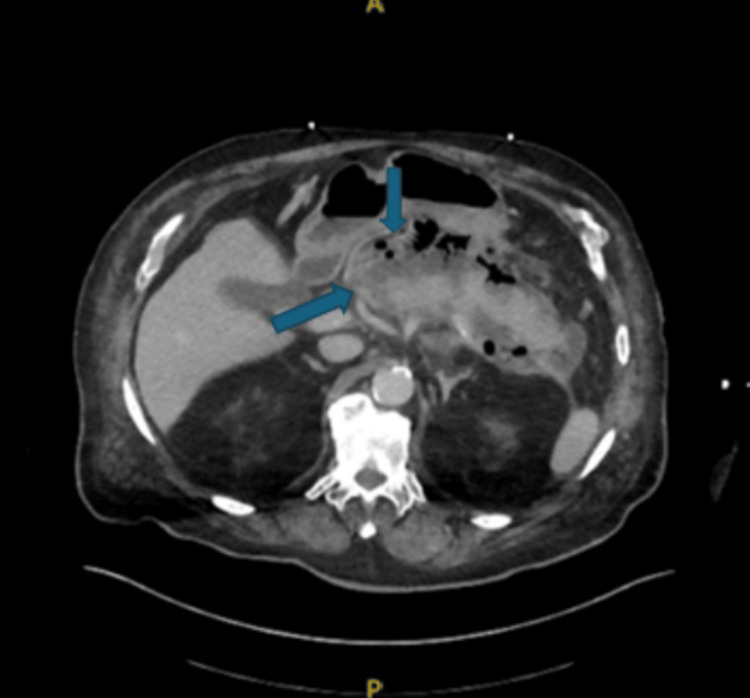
CT angiography of the abdomen and pelvis with and without contrast (axial view) showing multifocal areas of pancreatic necrosis: peripancreatic gas-containing fluid collection measuring up to 18.5 cm with the fluid collection abutting the greater curvature of the stomach and proximal small bowel loops CT: computed tomography
